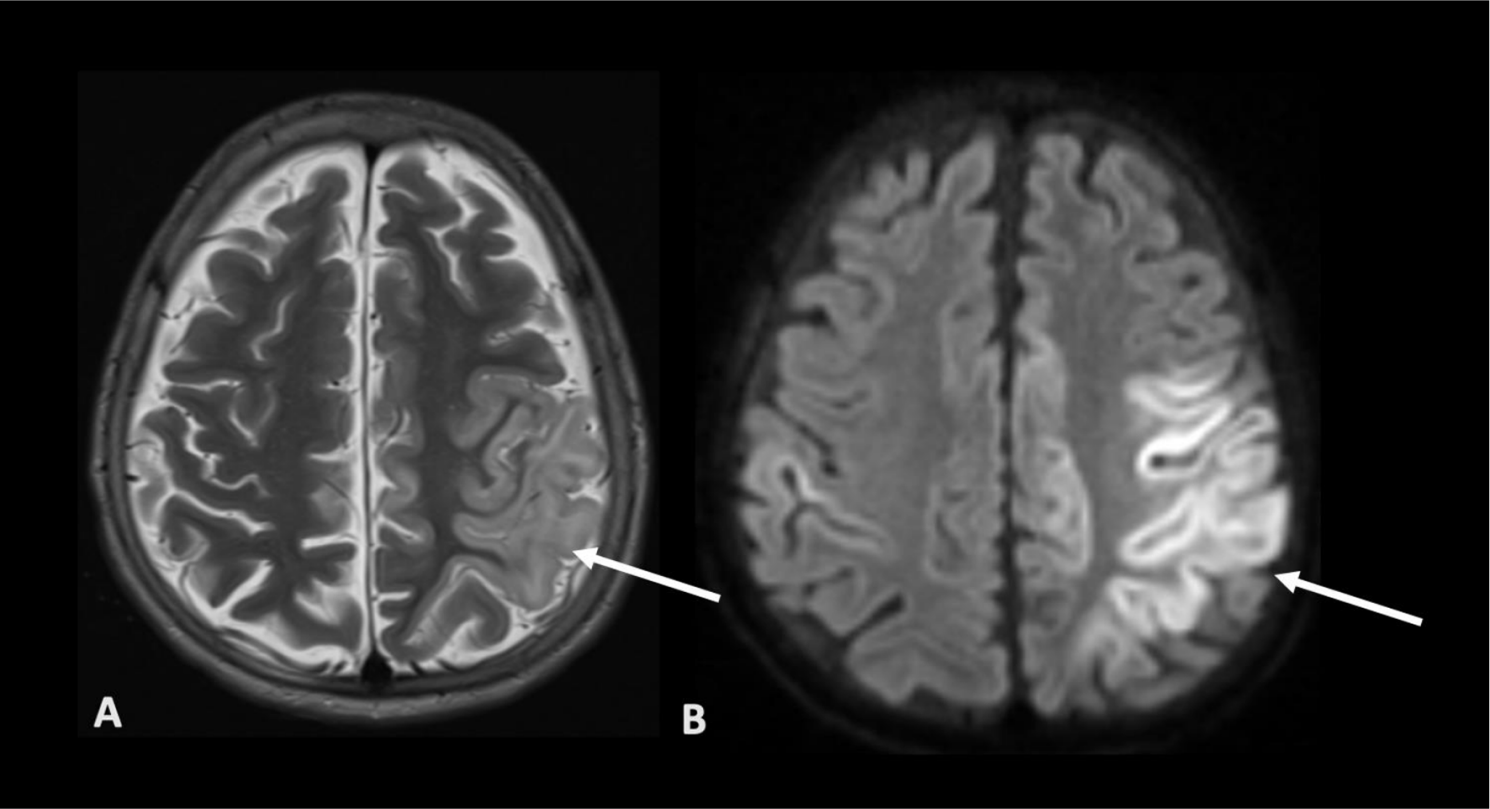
MRI brain images of P12 demonstrating features of generalised atrophy. Axial T2 weighted images (A) and axial diffusion weighted images (B) show extensive cortical signal change diffusion restriction and associated swelling in left posterior frontal and parietal regions. Similar changes were also present in the right hemisphere and lentiform nucleus. MRI – magnetic resonance imaging
